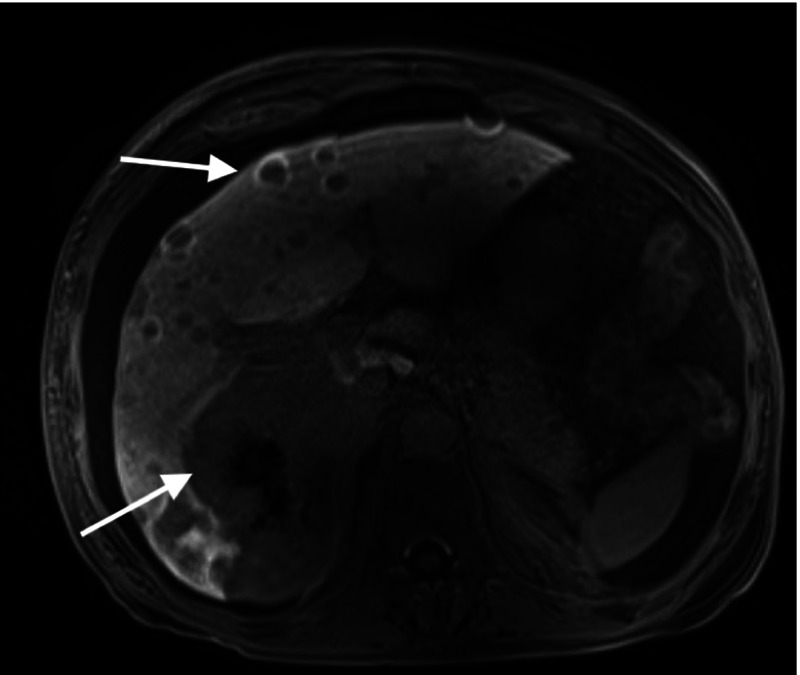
MRI of the liver prior to transplant. Arrows are depicting multiple enhancing liver masses.
